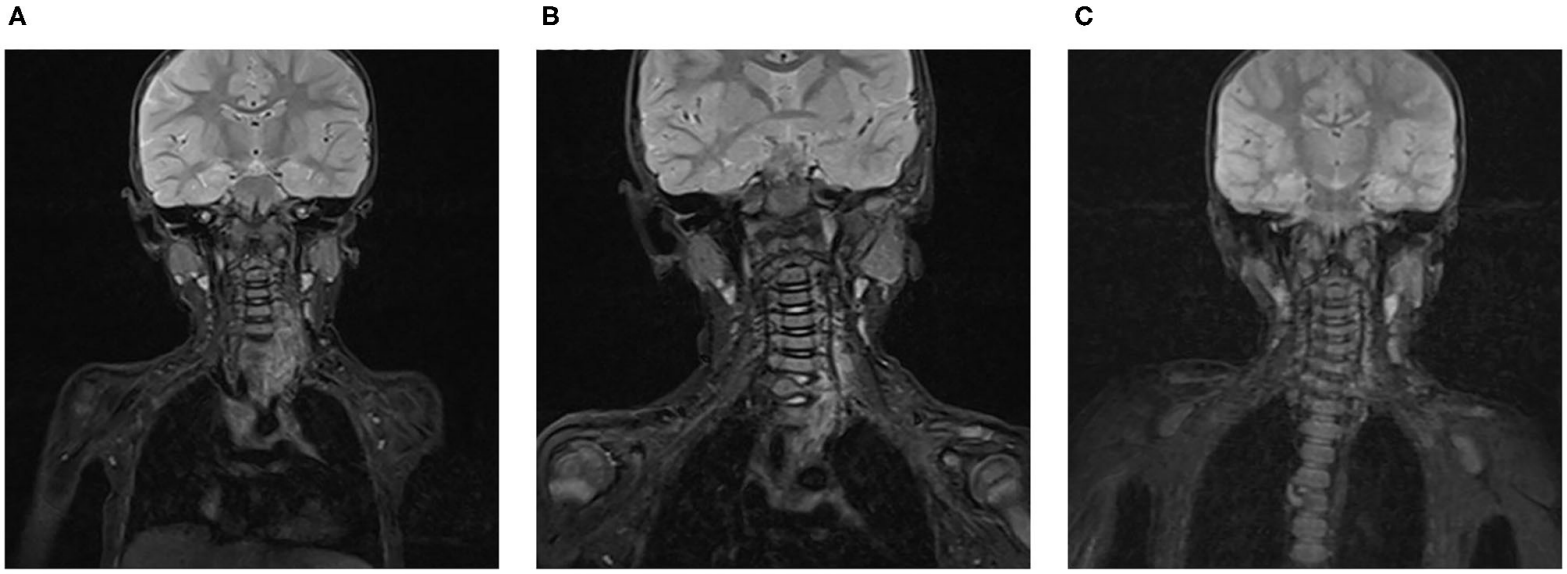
KHE in Patient 3: On MRI, a soft tissue mass in the left root of the neck can be seen before treatment (A). After 6 months (B) and 1 year (C) of sirolimus treatment, the size of the lesion was gradually reduced.
